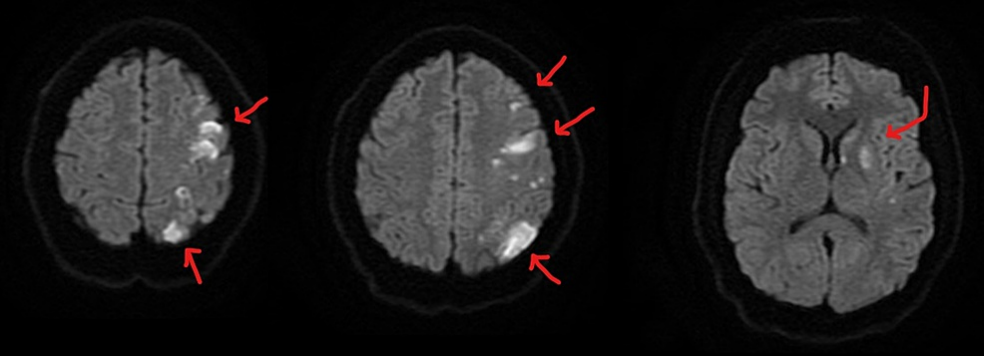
Patient's head MRI revealed multifocal acute infarcts – largest in the left parietal lobe measuring 2.7 × 1.4 cm and the left frontoparietal region measuring 2.6 × 1.6 cm, besides multiple smaller infarcts in the left basal ganglia, genu of the left internal capsule, and left frontal and parietal lobes.
